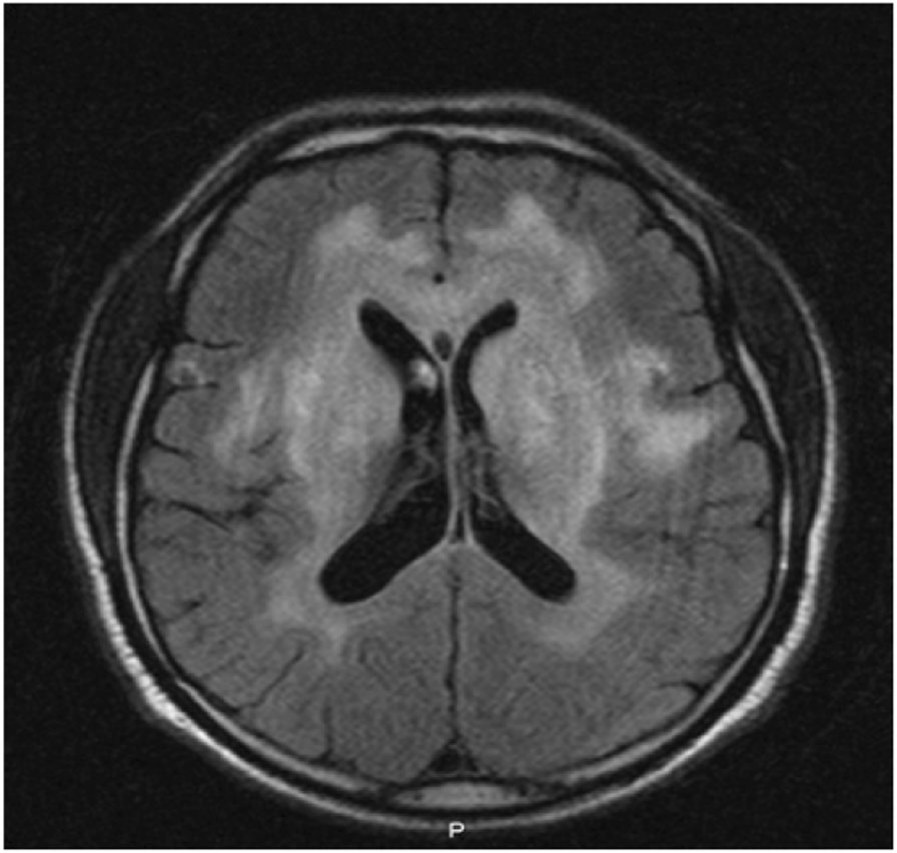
Magnetic resonance imaging shows confluent fluid-attenuated inversion recovery white matter signal involving supratentorial brain
